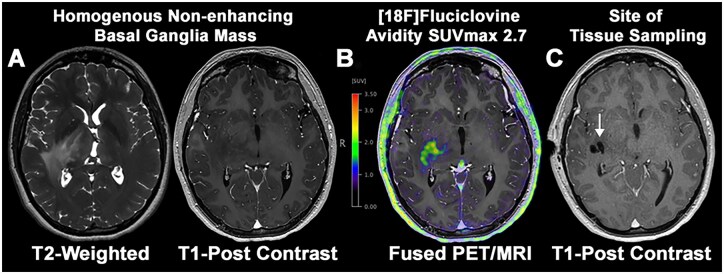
Amino acid [F18]Fluciclovine PET/MRI guides surgical tissue sampling. Multidisciplinary case review provided concern for primary glial neoplasm as a causative etiology of the non-enhancing lesion. This consensus opinion prompted the need for surgical tissue sampling as the next step in the patient’s care. However, the relatively homogenous MRI appearance of the non-enhancing lesion did not provide a definitive target. (A) Follow-up amino acid [F18]Fluciclovine PET/MRI surveillance imaging was performed 6 months after presentation to assess for elevated metabolism that could serve as a biopsy target. This demonstrated non-enhancing disease progression. (B) Elevated [F18]Fluciclovine avidity with an SUVmax of 2.7 was observed within the right basal ganglia that extended into the temporal stem. The [F18]Fluciclovine PET/MRI was integrated into the neuronavigational software and utilized to guide selection of a suitable tissue sampling target. (C) Post-surgical MRI demonstrates site of diagnostic tissue sampling corresponding to site of elevated [F18]Fluciclovine avidity (arrow).
